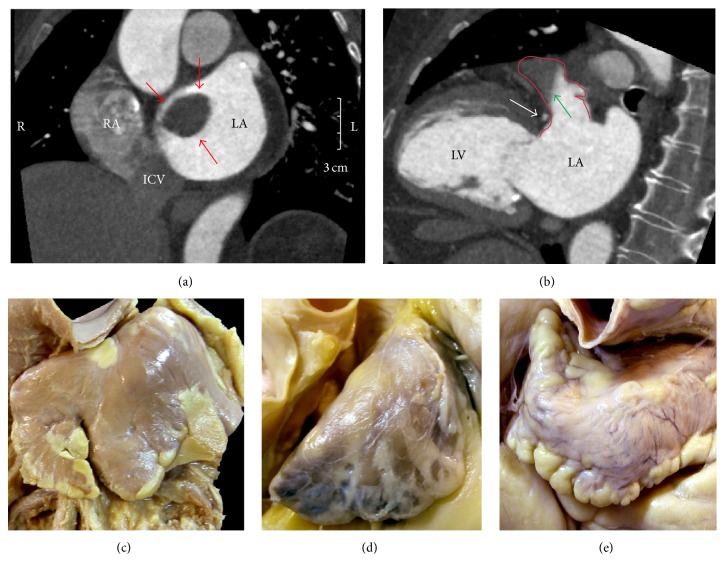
(a) Short-axis view of CT angiography showing a large clot (red arrows) in the left atrium attaching to the interatrial septum in a patient with atrial fibrillation. (b) Long axis view of the left heart showing the left atrial appendage (bordered in red) containing fluid-fluid level of unmixed contrast (green arrow) due to incomplete contraction of the left atrial appendage in this patient with atrial fibrillation. Note close anatomic course of the left circumflex artery (white arrow) near the neck of the left atrial appendage. (c, d, e) Images demonstrating significant interindividual variation in left atrial appendage morphology ((c) chicken wing; (d) cauliflower; and (e) windsock). ICV = inferior cava vein, LA = left atrium, LAA = left atrial appendage, LV = left ventricle, and RA = right atrium.
